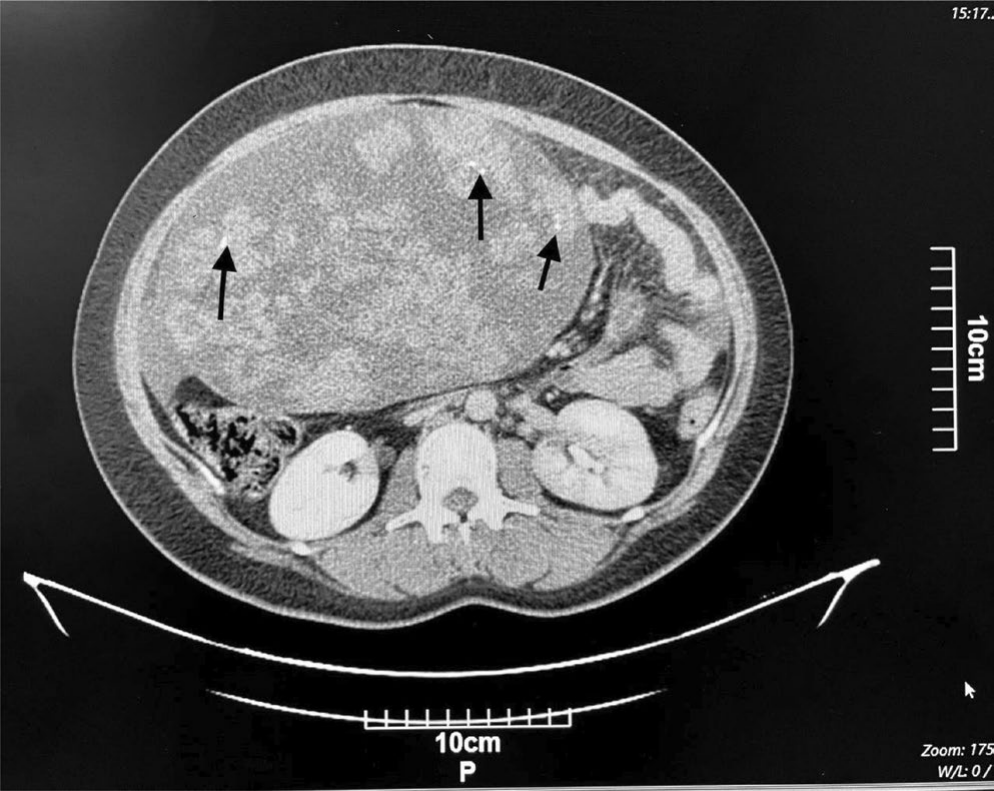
CT scan of the abdomen and pelvis (transverse view) showing solid cystic mass with calcification (black arrows).
